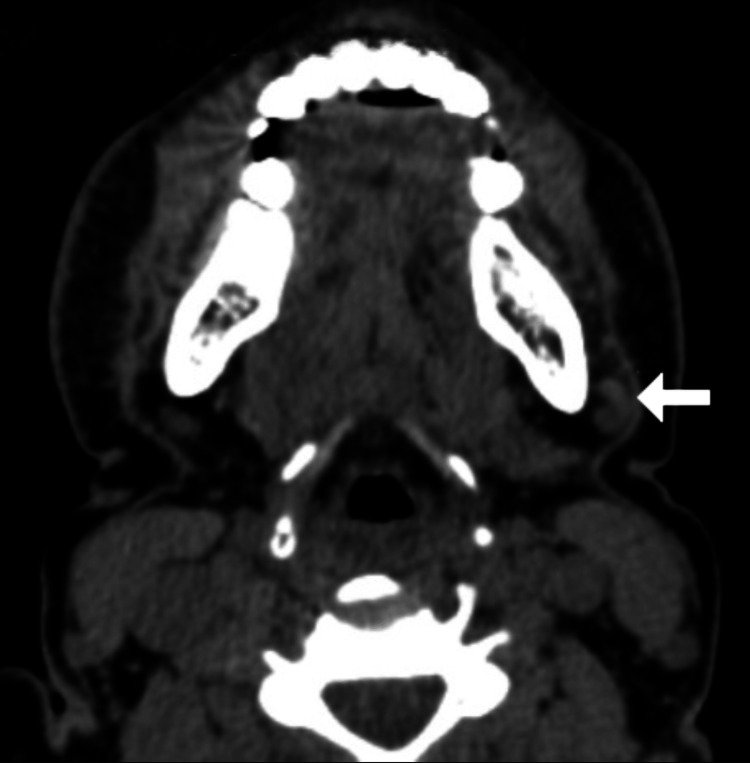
CT of the head and neck (axial view) showing multiple cervical lymph nodes (white arrow) CT: computed tomography
